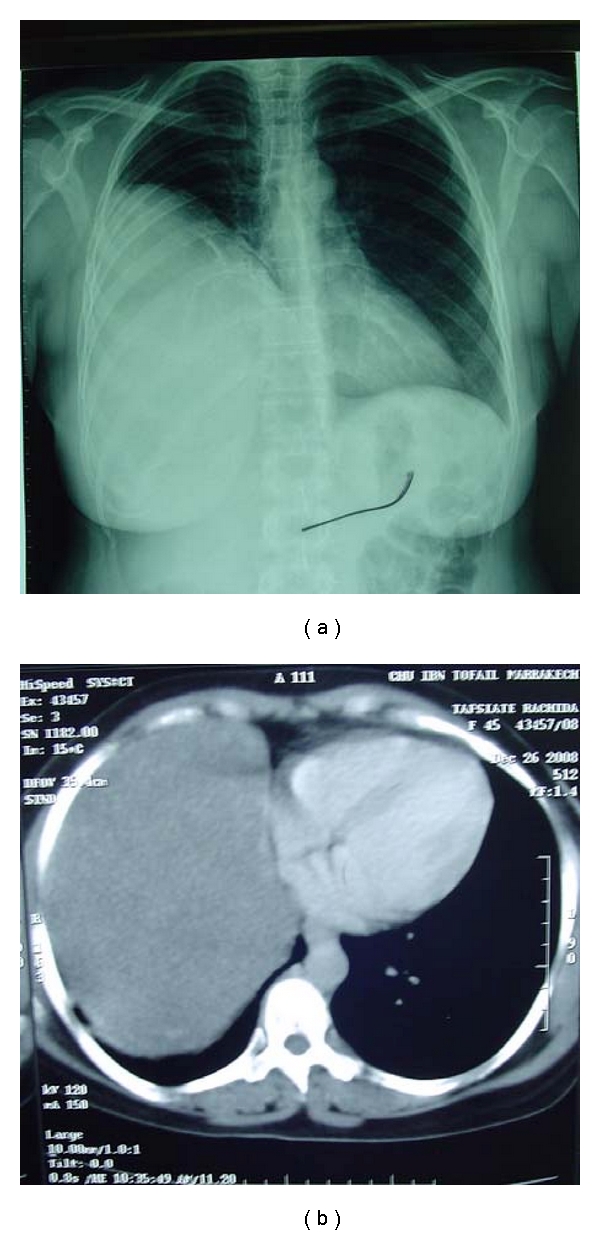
(a) Initial chest radiography showed right basithoracic mass with clear limits and obtuse connection angles. (b) Initial computed tomogram with contrast revealed one mild homogeneous, well-defined mass that compressed the mediastinum to left side.
